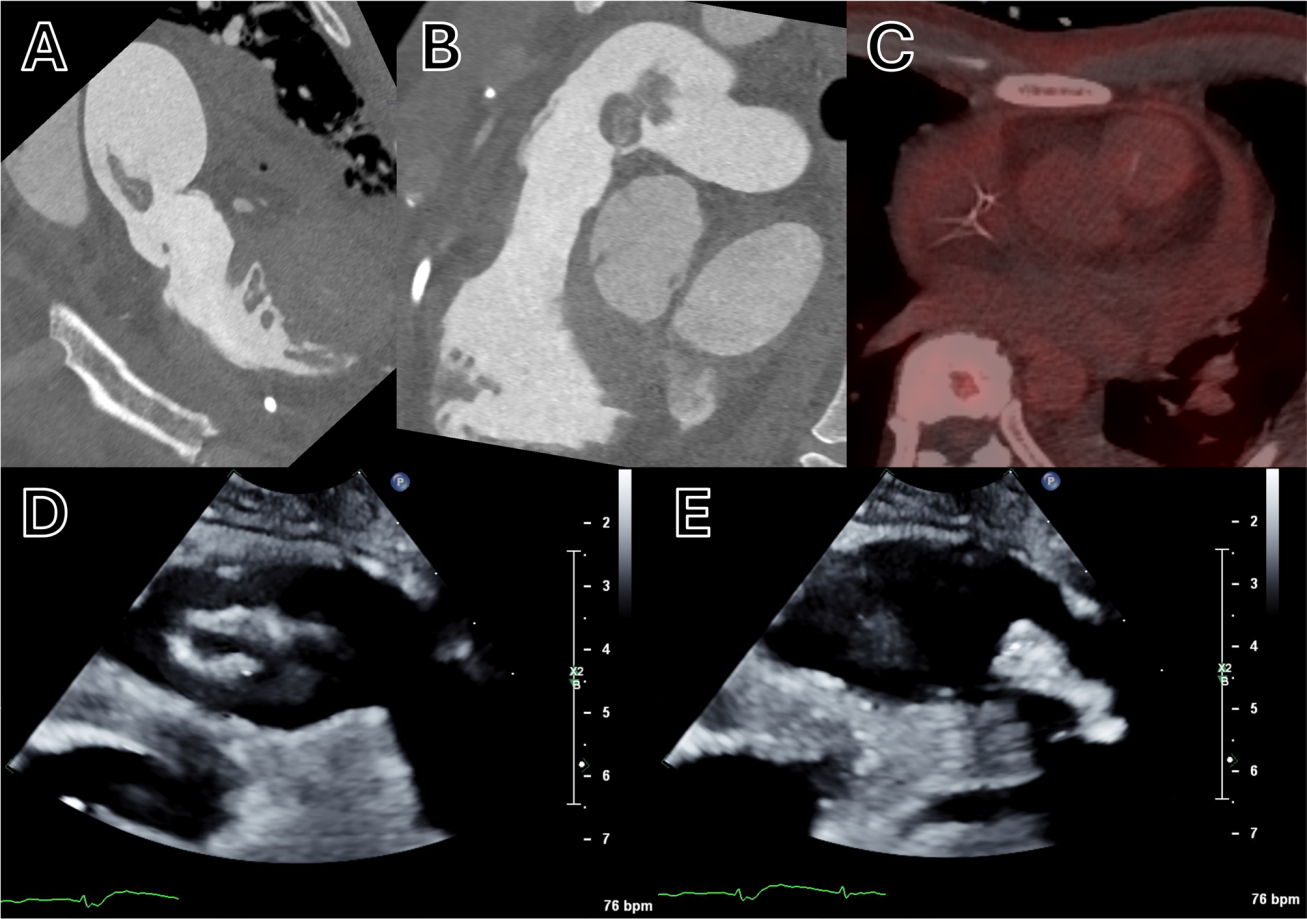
A + B CT angiography with two perpendicular cross-sectional reconstructions of the RVOT demonstrating destruction of the PV and a large mobile vegetation. C PET CT without hypermetabolic activity on the PV. D + E TTE in parasternal short-axis of the RVOT demonstrating vegetation motion in diastole and systole
